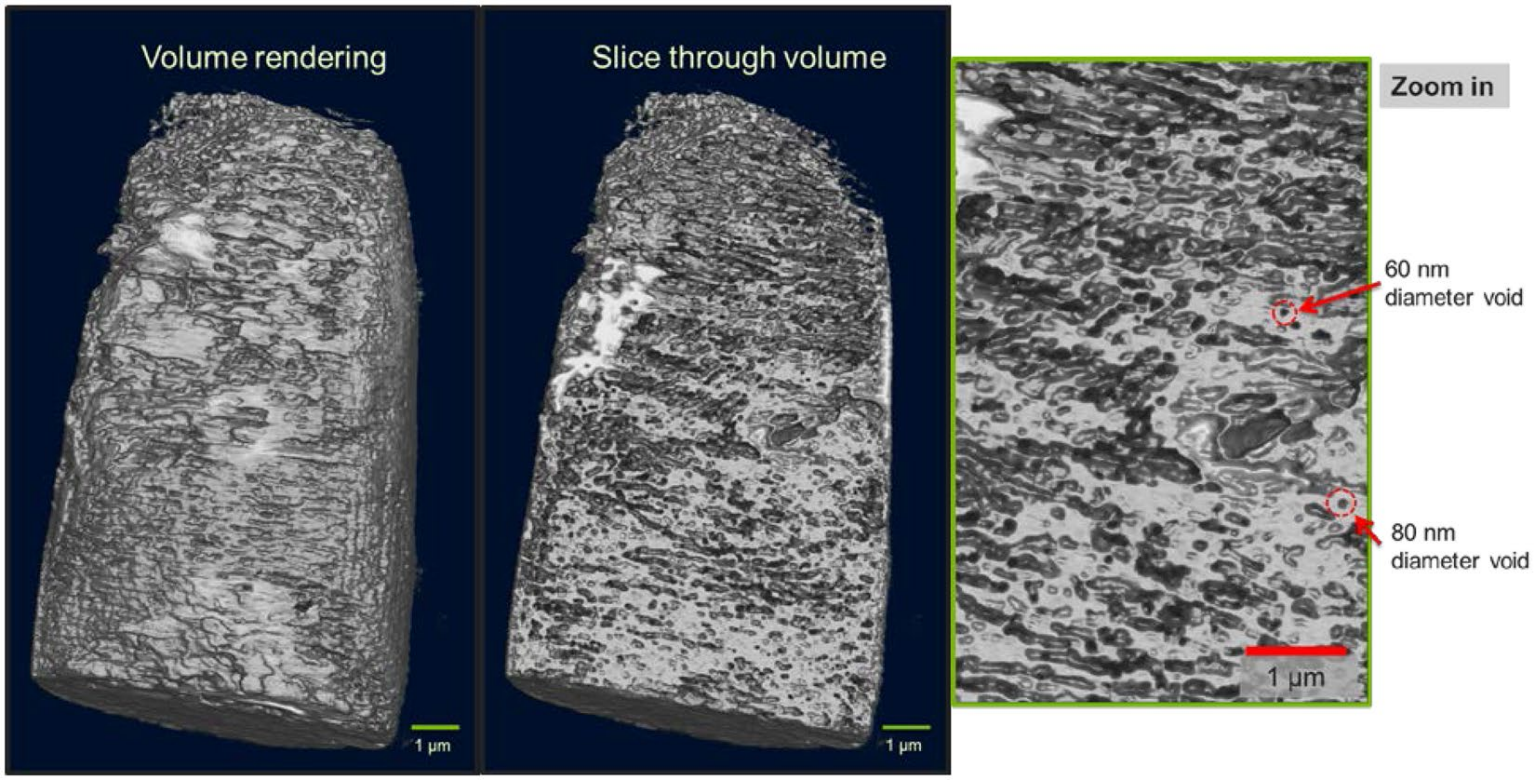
X-ray tomography imaging of tooth sample using SiS ZnO as contrast enhancement. Reconstruction results showing sequence of total volume rendering, a slice through the volume, and a zoom into the section of the slice highlighting the presence of detailed nanoscale features and well-defined pores as small as 60 nm to 80 nm in diameter within the tooth.
